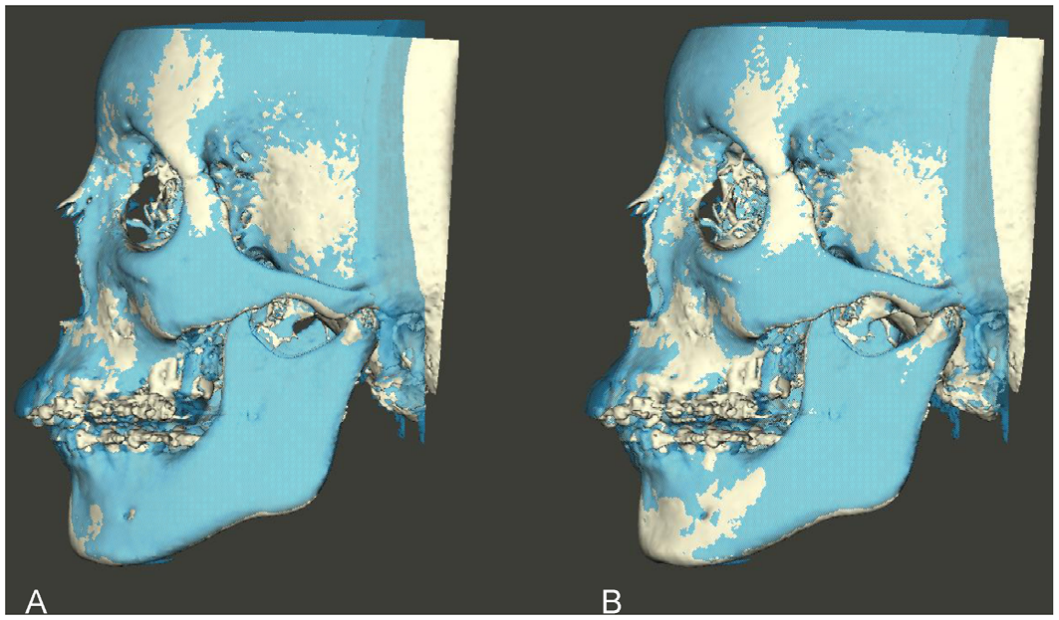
Transparency overlay of superimposed 3D CBCT models. Left side view. (A) models registered on the anterior cranial base. (B) same models registered on the left zygomatic arch.
